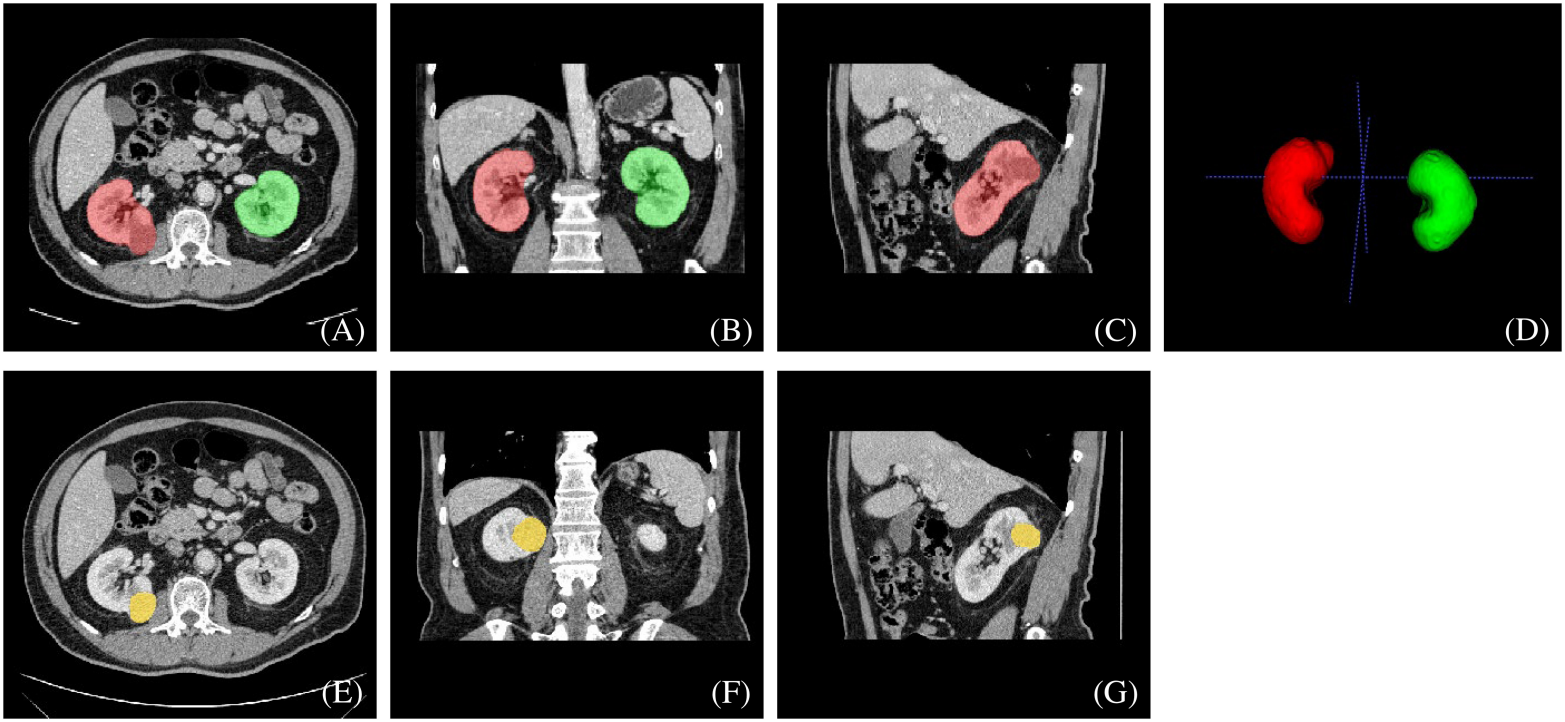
An example of manually segmented kidneys and renal mass from CT images. We used different mask colors to delineate different parts of the cross-section image: (A–D) red mask=right kidney, green mask=left kidney, (E–G) yellow mask=neoplasm.
